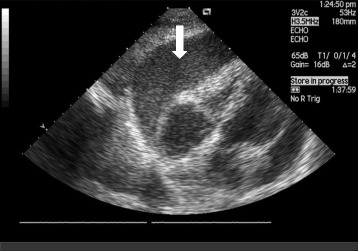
Echocardiography: hyperechogenic fluid around the whole heart (*arrow*)
